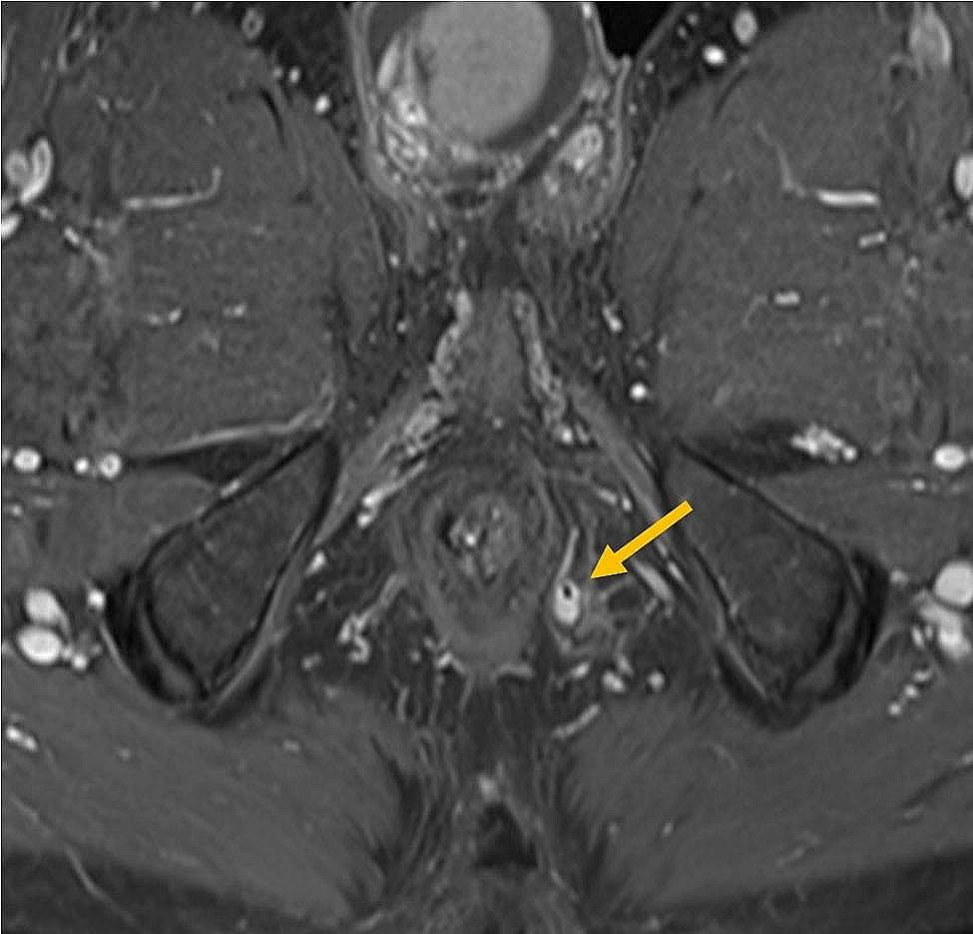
a. Pre-treatment MRI: An axial T1-weighted sequence with fat suppression after contrast administration with a total MAGNIFI-CD score of 18 based on a complex fistula longer than 5 cm with intralevatoric extension, pronounced T1 hyperintensity and for more than 50% consisting of granulation tissue. The active fistula is indicated with an orange arrow
